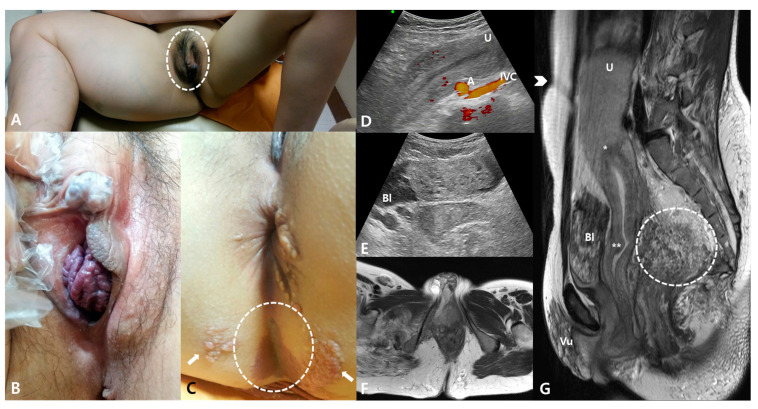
(A) Hemihypertrophy of the right lower limb, hemangioma of the right vulva, and ambiguous genitalia with an elongated clitoral hood, approximately 4 cm in length (shown in the dotted circle). (B) An enlarged, 2.0 × 1.8-cm^2^ whitish globular clitoris glans and a purplish bulging anterior vaginal wall. (C) Typical port-wine nevus (dotted circle) and bullous plaques (arrows) on the buttock. (D) The upper margin of the uterine fundus (U) was noted around the abdominal aorta (A) and inferior vena cava (IVC) on sonography. (E) A typical sponge-like shadow consisting of a mass-like lesion without Doppler flow in the bladder (Bl) was observed by sonography. (F) Hemihypertrophy of the right lower limb and hemangioma of the right vulva with an enlarged clitoris viewed with MRI. (G) A typical sponge-like shadow in the bladder (Bl) and rectum (dotted circle) viewed in T1-weighted images, and an extensive cavernous hemangioma in the pelvis pushing the uterine fundus (U) up to above the umbilicus (arrowhead) shown on MRI. * (internal cervical os);  (external cervical os).
